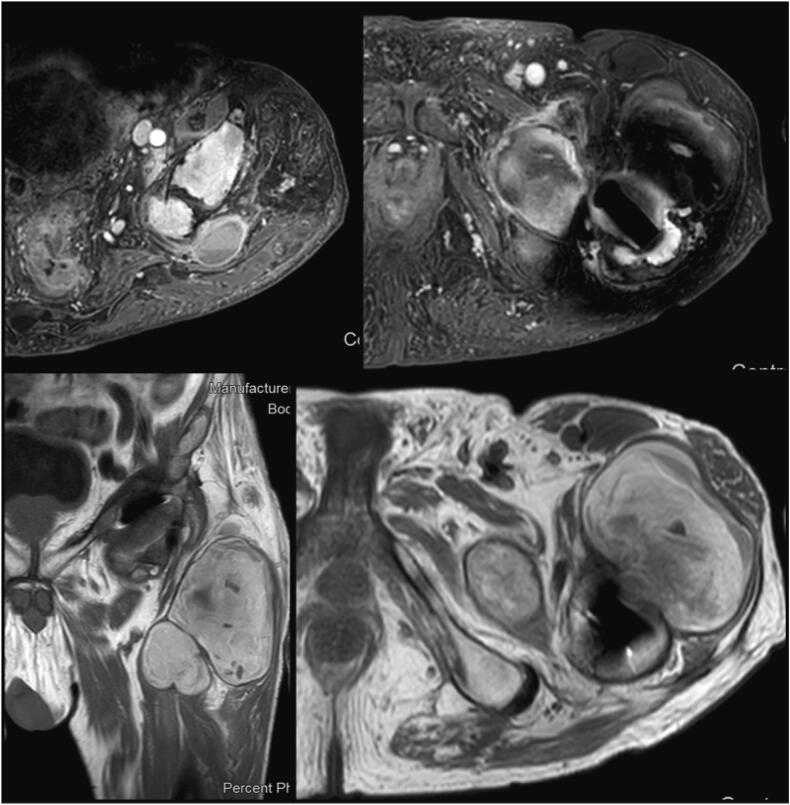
Preoperative MRI, Adverse Local Tissue Reaction and pseudotumor - bone resorption around the left femoral stem, left ilium and the acetabulum; protein/hemorrhagic subsolid collection of 7 cm in diameter inside the proximal part of the quadriceps muscle, which involved also the iliopsoas bursa and extended to the pelvis.
